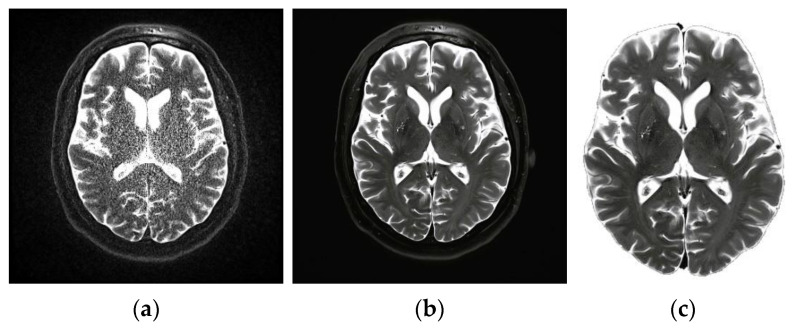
(a) Noisy MRI image, (b) filtered image, (c) and output of skull elimination.
